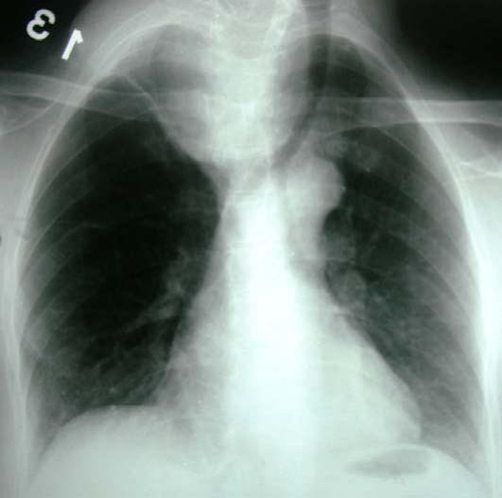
Chest radiography reveal a large soft tissue mass occupying the superior right hemithorax.
